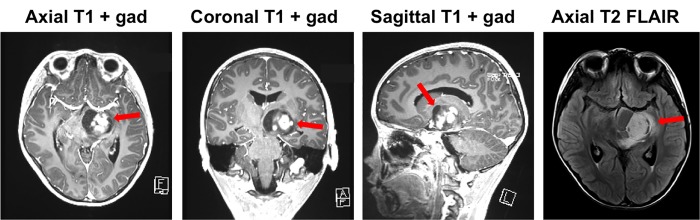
Preoperative magnetic resonance imaging (MRI). Preoperative MRI showing the tumor (red arrow) in the left thalamus/midbrain. The FLAIR (fluid-attenuated inversion recovery) image demonstrates mild peritumoral edema, gad: gadolinium.
